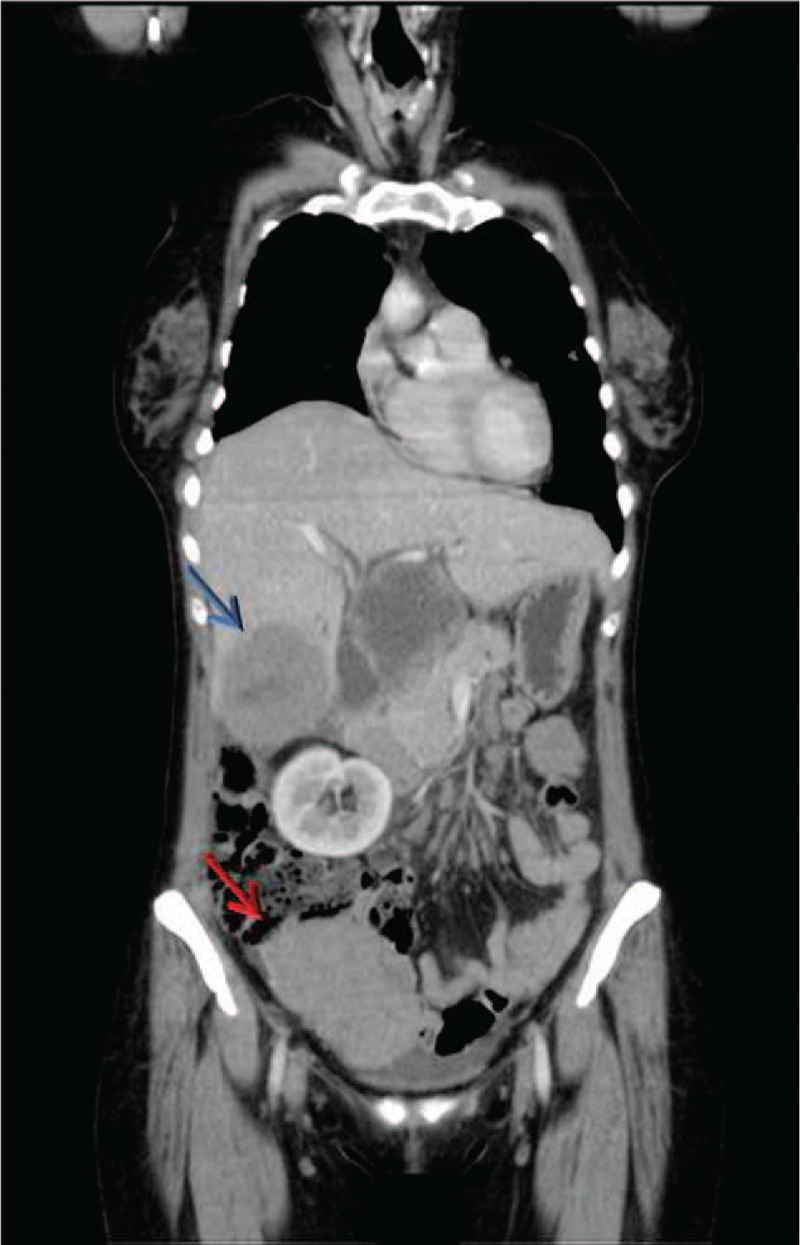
Enhanced-CT coronal section showed an increase in size of right abdominal retro-peritoneal lesion (red arrow) and peri-hepatic hilus lymphnodes (blue arrow) with necrotic central area and significant compression of the adjacent structures.
